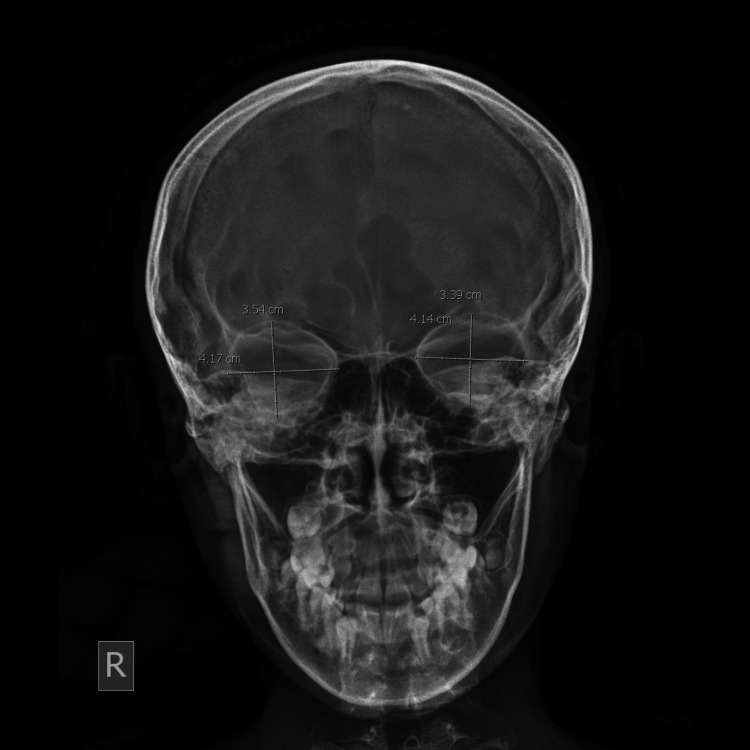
Frontal digital radiograph of skull showing the orbital margin and measurement of orbital height and width.
